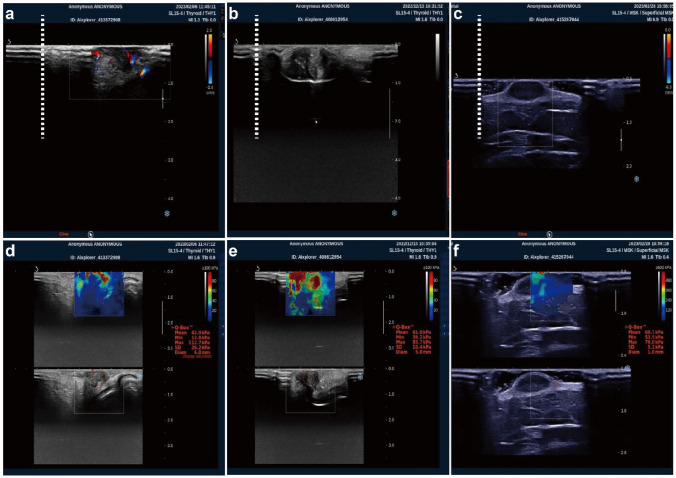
Ultrasound findings of keloid. a–c Keloid visualization. d–f Keloid blood flow visualization
